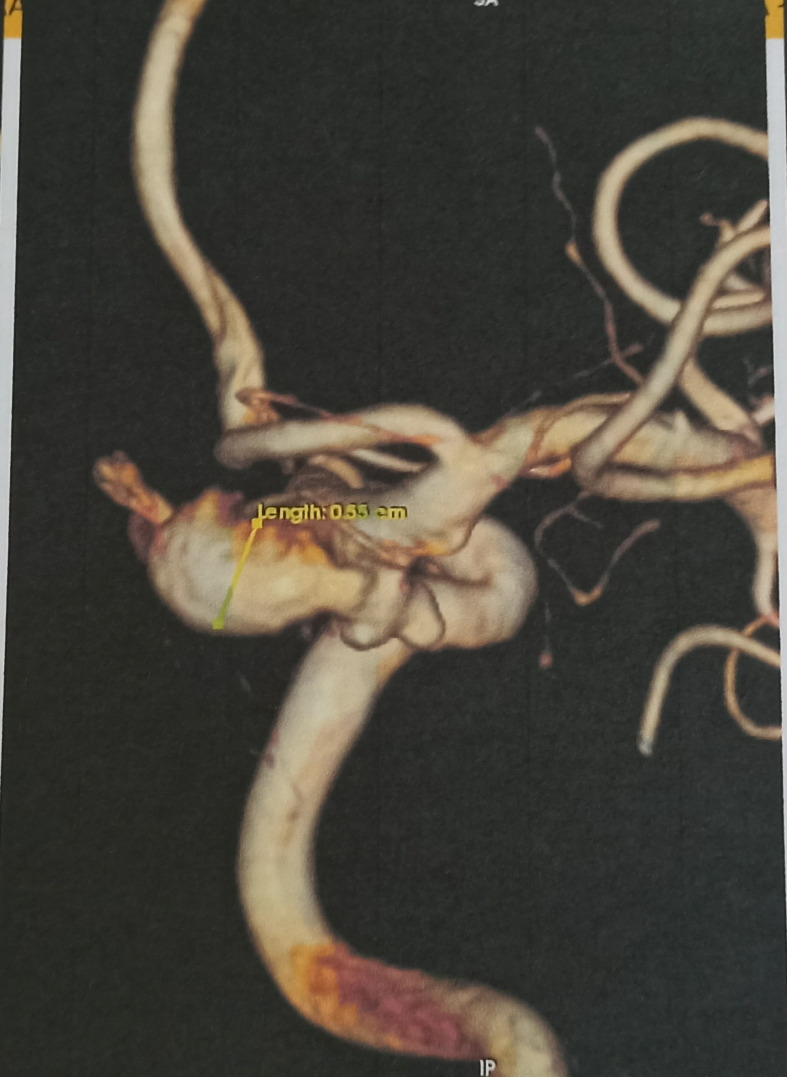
3D CT angiography found the size of aneurysm in the neck
